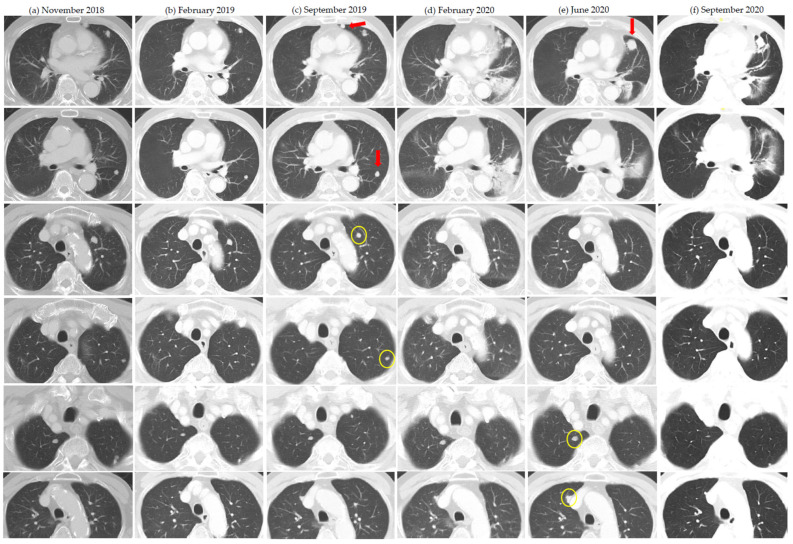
Serial abscopal responses in an 84 year-old man receiving second-line nivolumab for metastatic renal cell carcinoma since May 2018. (a–f) Serial CT scans of lung metastases from November 2018 to September 2020. Red arrows indicate lesions that received radiation. Panel (c) lesions received stereotactic body radiation therapy at 42Gy in five fractions (anterior lesion) and 54Gy in three fractions (posterior lesion), both in October 2019. Panel (e) lesion received 25Gy in five fractions in July 2020. Yellow circles indicate out-of-field lesions that showed abscopal pattern of response.
